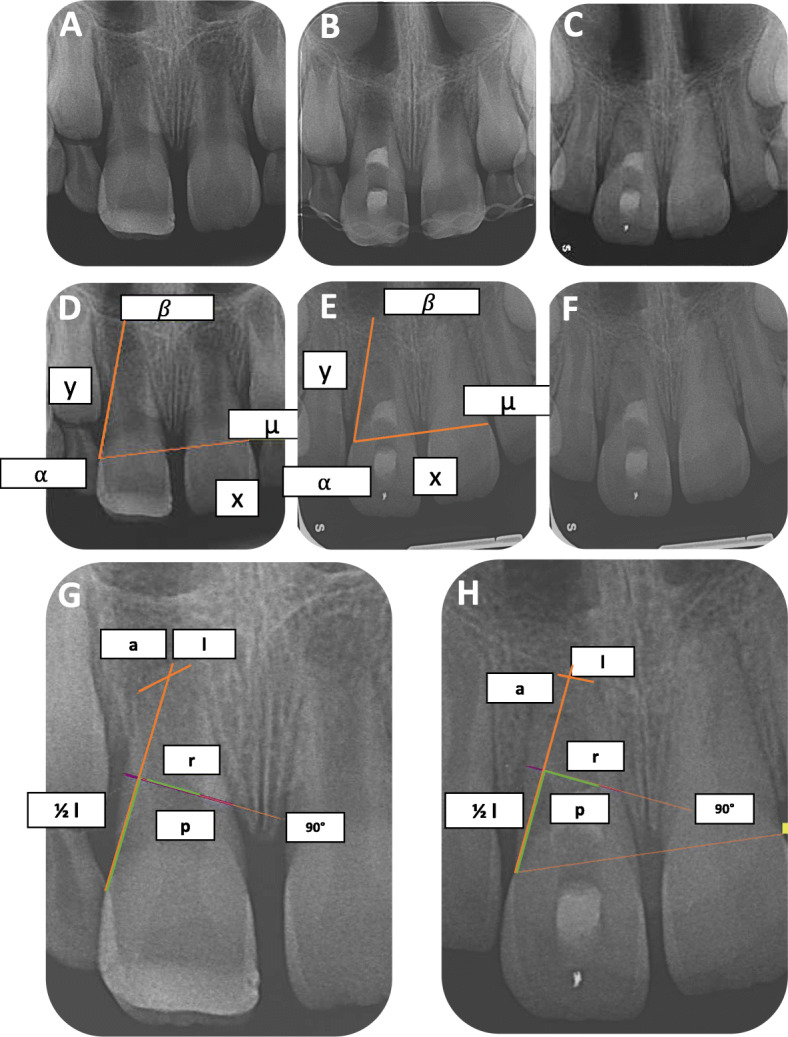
A representative case:*A Preoperative radiograph of a necrotic, immature teeth with an open apex of an 8-year-old girl. B Radiograph directly after the treatment to check the coronal MTA plug. C Postoperative radiograph after 10-month follow-up period showing disappearance of periapical radiolucency, apical closure and distal sign of resorption at the coronal third of the root. Selected landmarks (α, β, μ) on the preoperative (D) and postoperative radiograph (E) to adapt the postoperative radiograph and set the scale (x,y). F The corrected postoperative radiograph after using TurboReg plug-in application of ImageJ. G The measurement of the preoperative radiograph. H The measurement of the postoperative radiograph. The length (l) increased from 12.71 mm to 13.51 mm, the root thickness (r) increased from 6.12 mm to 6.27 mm and the pulp space (p) increased from 3.29 mm to 3.38 mm. The size of the apical diameter (a) decreased from 3.24 mm to 2.09 mm
